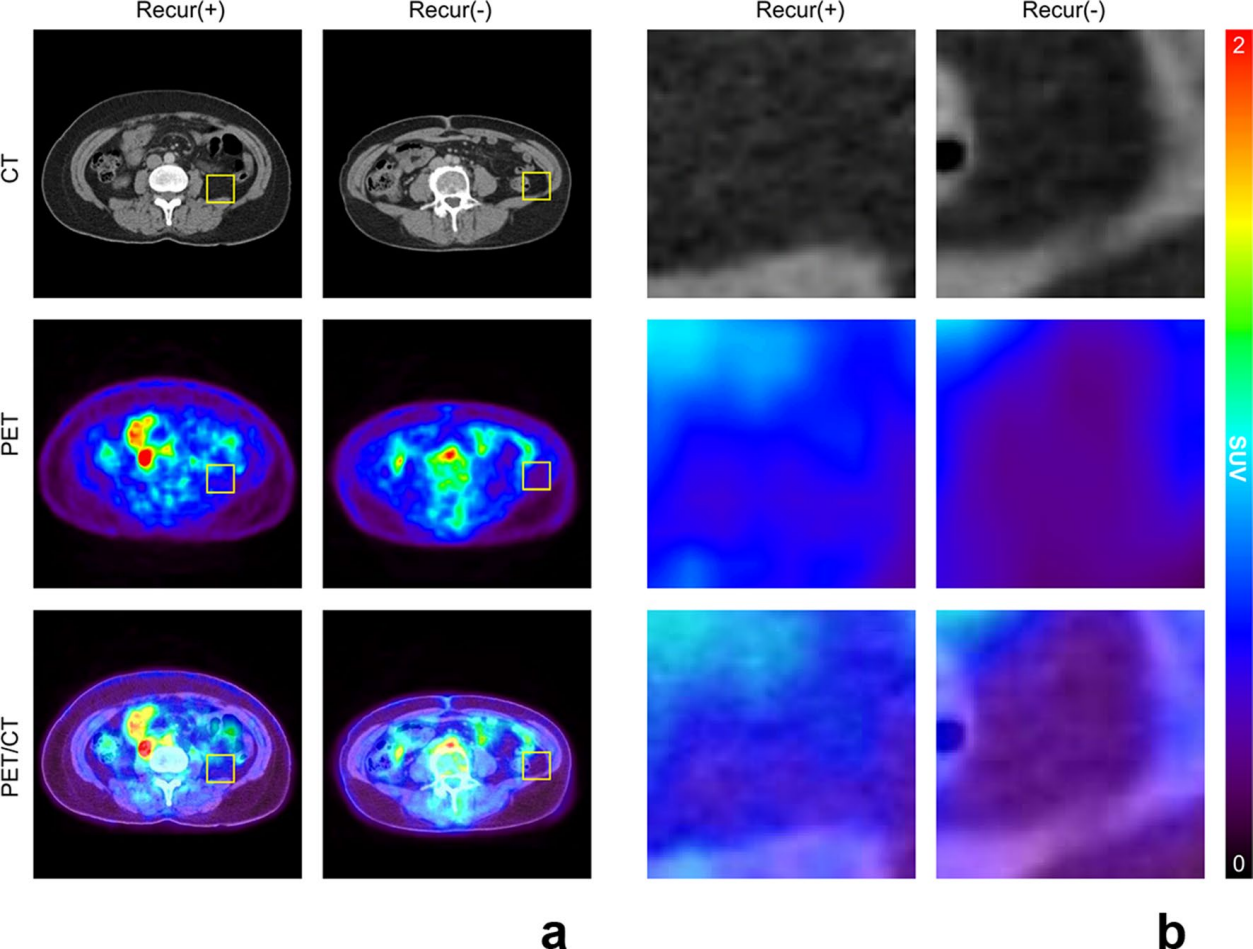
Representative images of (a) ^18^F-FDG uptake of visceral adipose tissue (VAT) according to the breast cancer recurrence status and (b) their corresponding magnified views. CT: computed tomography, PET: positron emission tomography, Recur: Recurrence.
